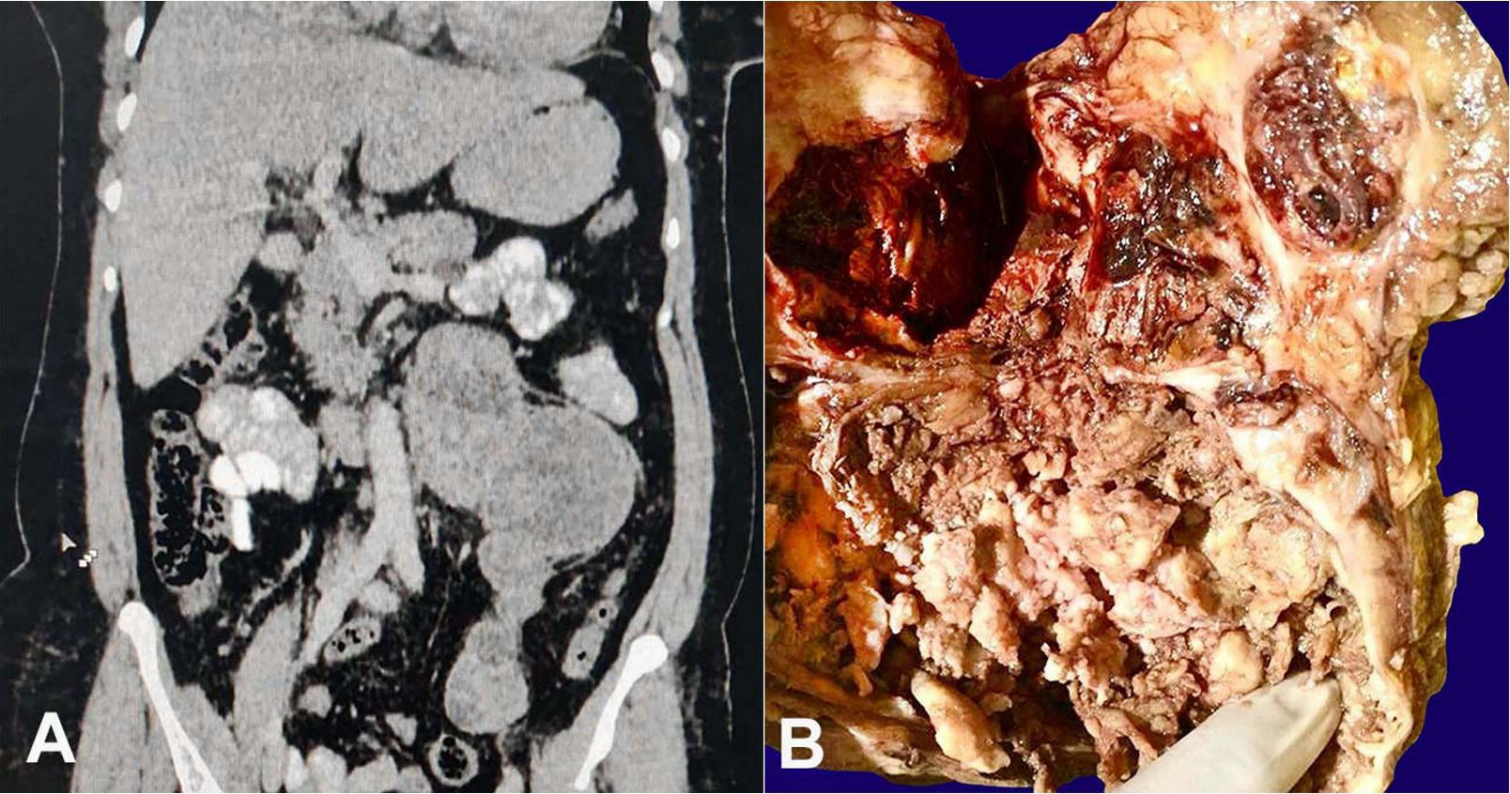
A – Computed tomography scan (coronal view) showing a longitudinally oriented, heterogeneously enhancing solid cystic retroperitoneal mass; B – Gross view of the solid cystic mass with areas of hemorrhage and necrosis. Note clotted blood within the cysts.
